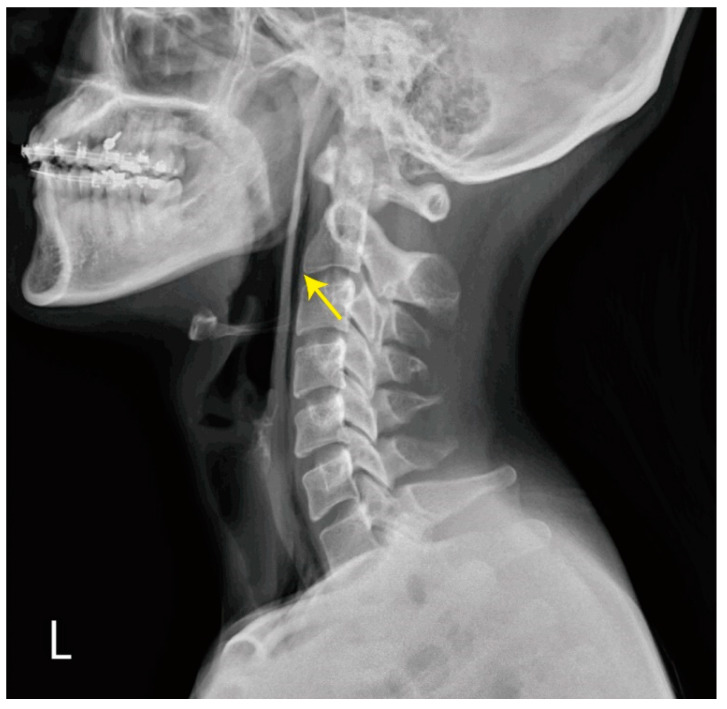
Chest X-ray showed subcutaneous emphysema over the neck and gas outlining from neck extending into the mediastinum (yellow arrow).
